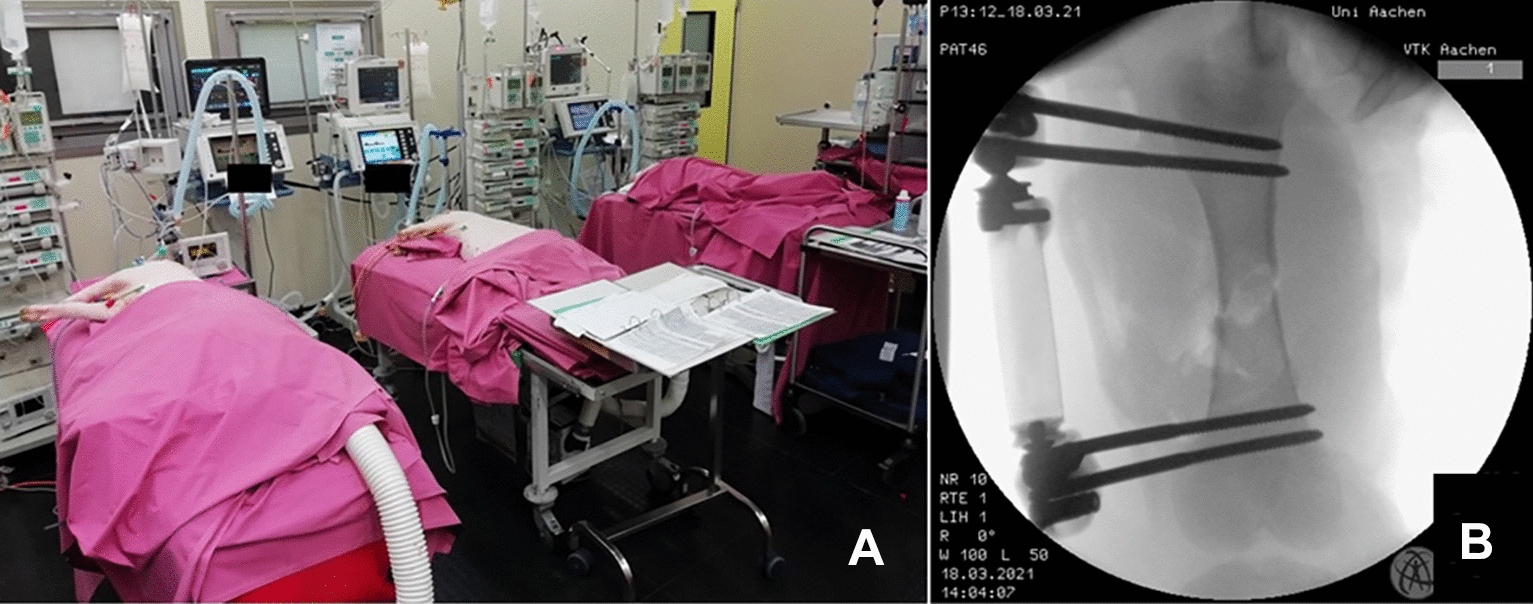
A Intensive care experimental setup. B C-arm X-ray confirming the diaphyseal tibia fracture
